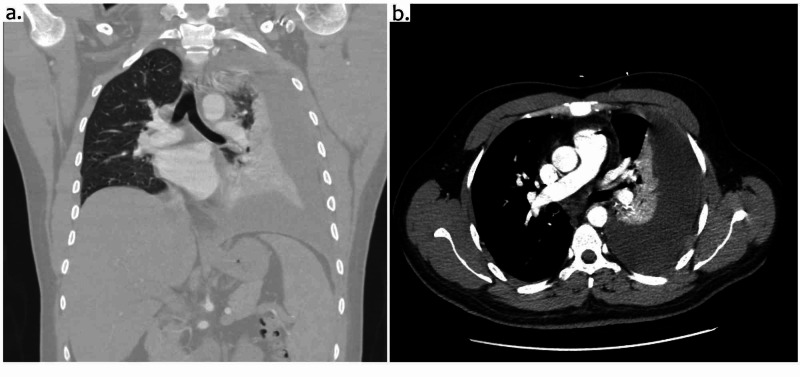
CT scan of the chest: coronal plane (a) and cross-sectional plane (b) showing large pleural effusion on the left side with atelectasis of the left lung.
